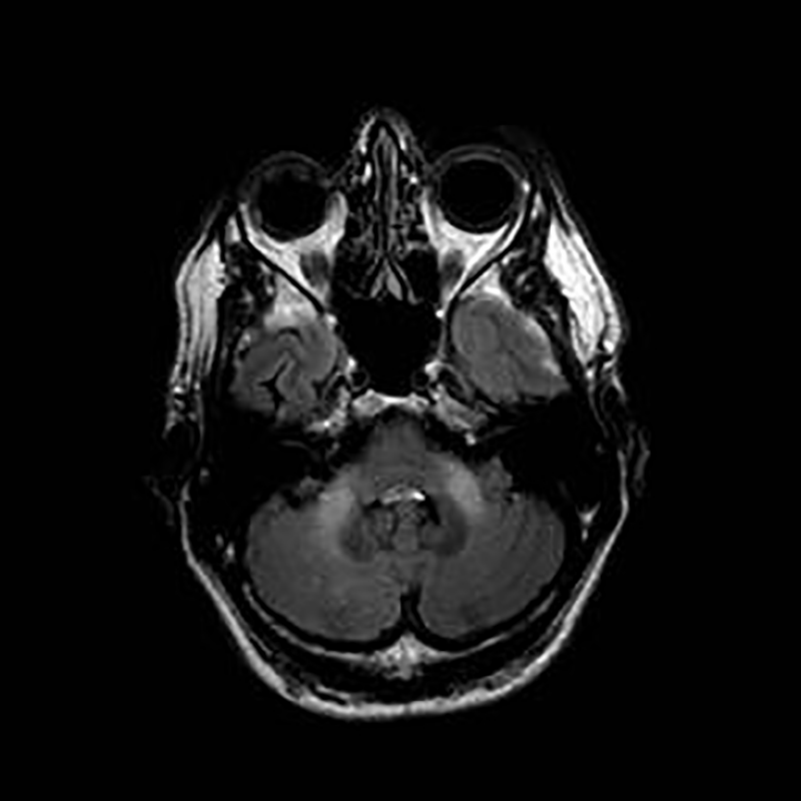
Axial T2-fluid-attenuated inversion recovery (FLAIR) image of the brain, showing high signal in the middle cerebellar peduncles, in a case of fragile X tremor-ataxia syndrome (FXTAS).
